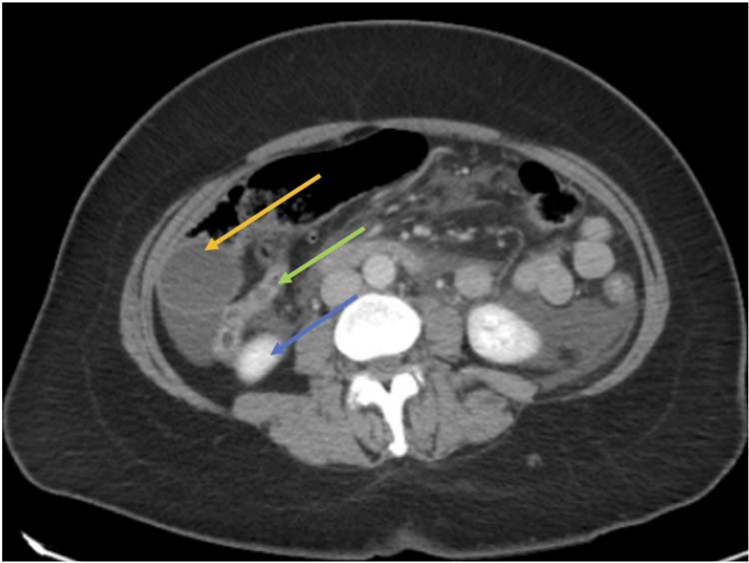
CT axial view showing the appendix (green arrow) inferior to the gallbladder (orange arrow) and superior to the right kidney (blue arrow).
